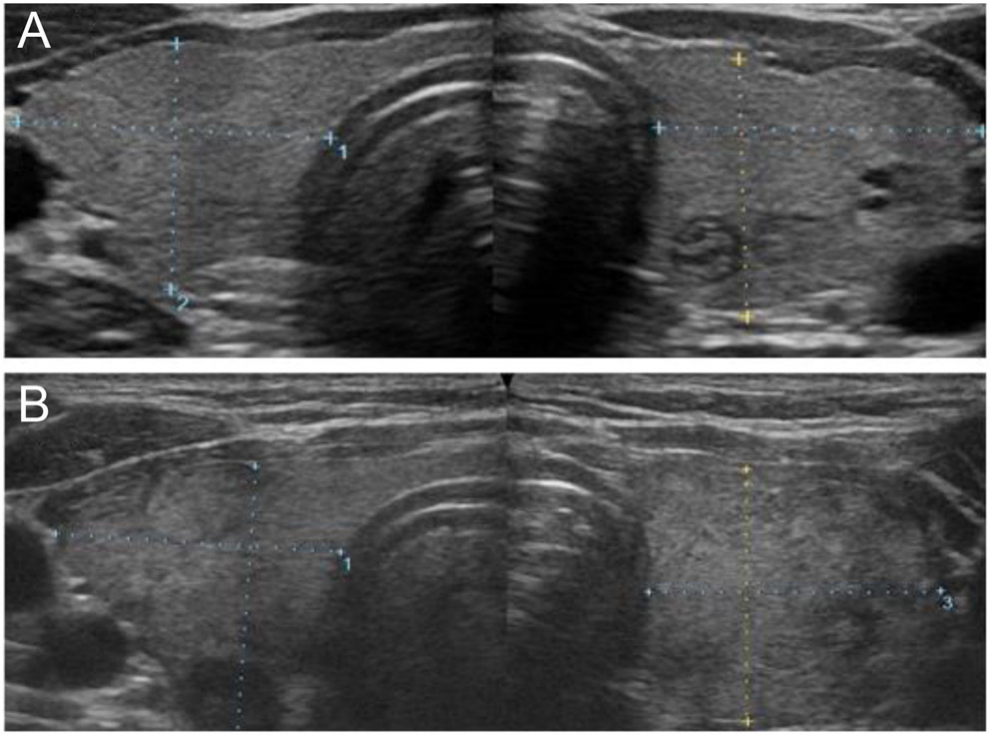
Ultrasonography of the thyroid gland. Ultrasonography of the thyroid gland at diagnosis of acromegaly (A) and admission for SARS-CoV-2 and influenza infection (B).
